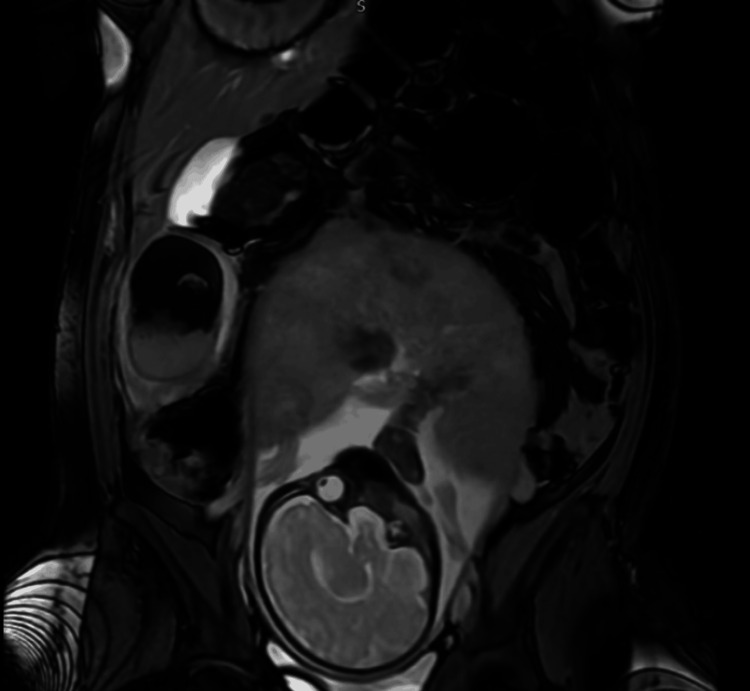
MRI showing the gravid uterus with the fetus and a right adnexal mass (dermoid cyst)
